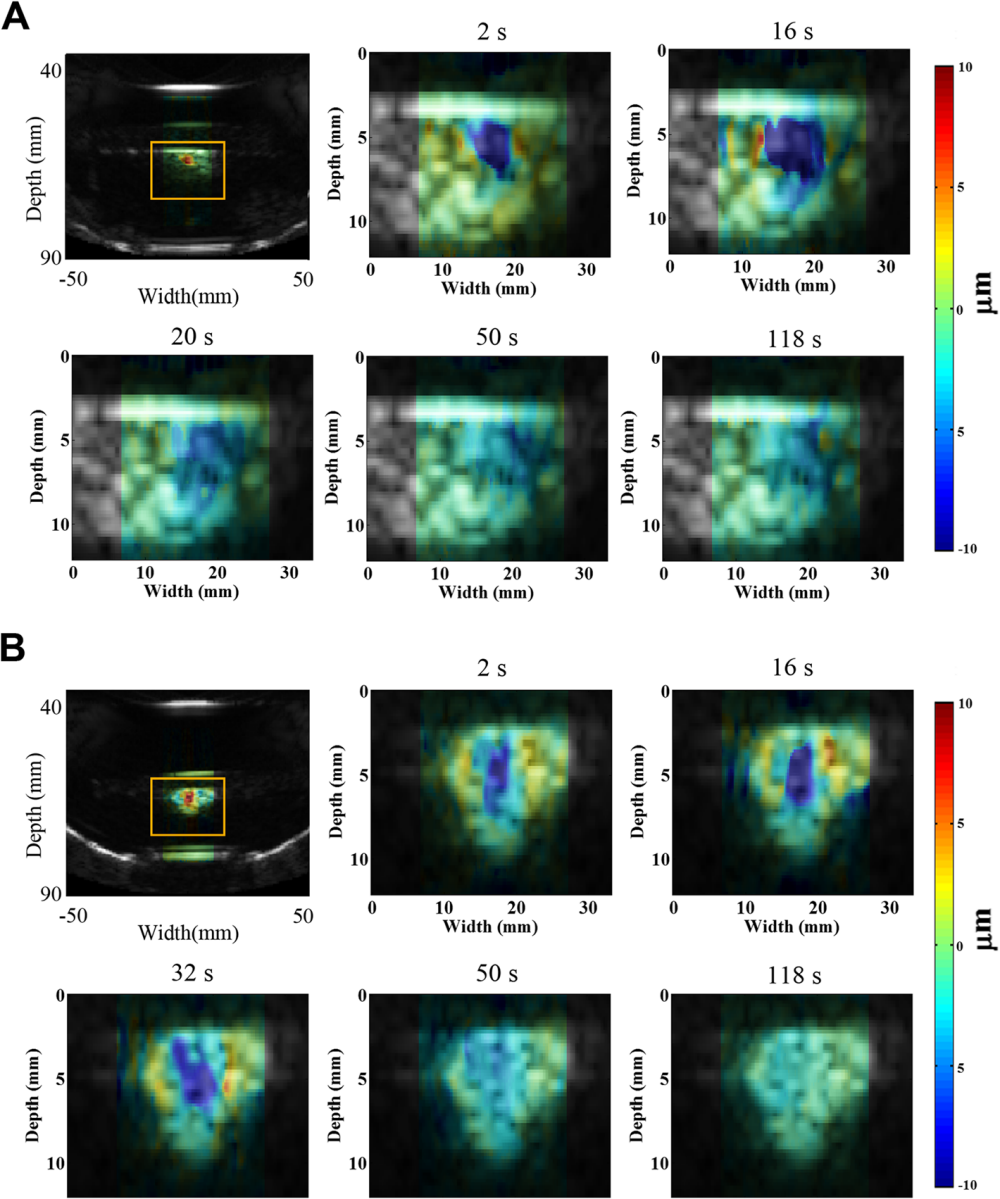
Harmonic motion imaging for focused ultrasound (HMIFU) ablation monitoring in 2D overlaying on B-mode images in (a) normal breast and (b) IDC tissue. Tissue motion during heating is denoted by alternating *red* and *blue. Red* represents the motion moving toward the transducer, and *blue* represents the motion moving away from the transducer. Peak negative harmonic motion imaging displacement frames during a 50-Hz cycle at five representative timepoints were selected from the HMIFU treatment monitoring sequence to show the decrease of focal displacement as the thermal lesion forms
